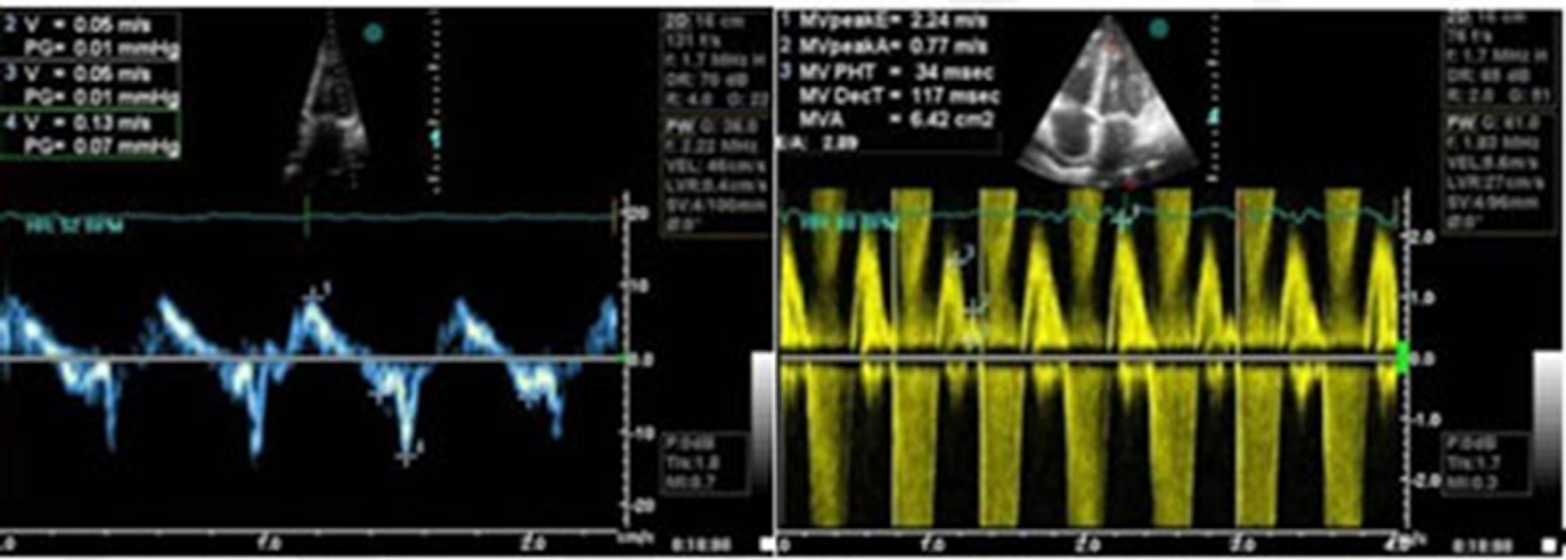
Pulse wave and tissue doppler study in echo showed diastolic dysfunction grade III
